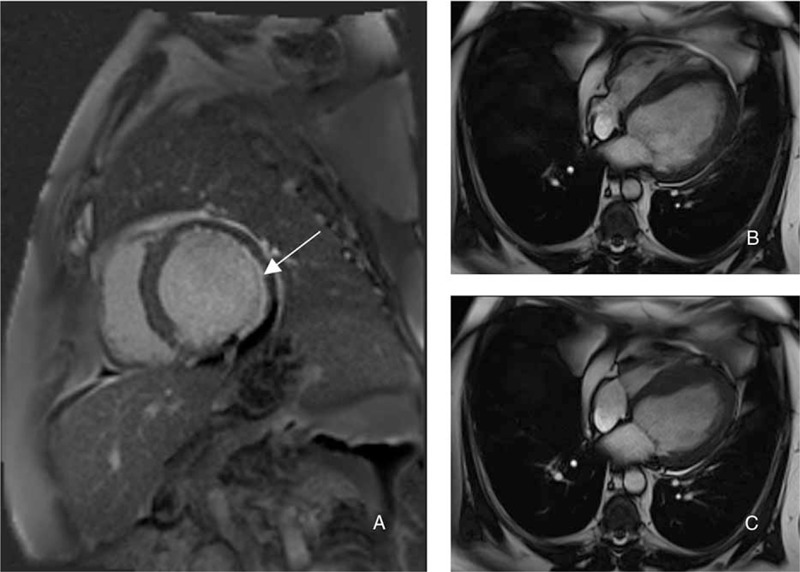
Cardiac magnetic resonance imaging. Late gadolinium enhanced image (A) showing a subendocardial posterolateral myocardial infarction. Cine-cardiac magnetic resonance acquisitions (diastole (B) and systole (C)) showing a severely impaired left ventricular function (LVEF estimated at 30%).
